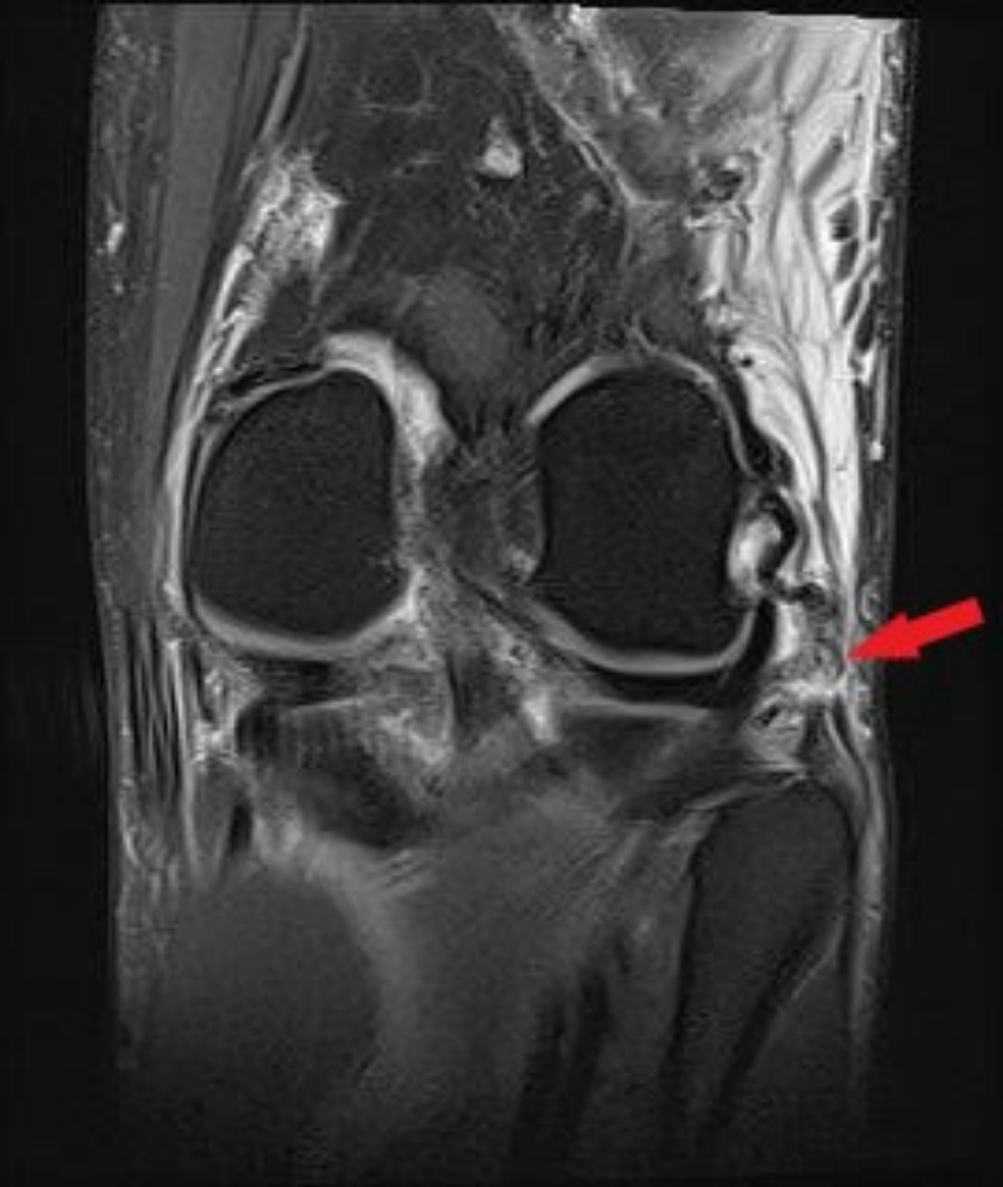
Coronal T2-weighted MRI of the left knee in patient 1 Coronal T2-weighted MRI of the left knee demonstrating LCL tear (red arrow).
